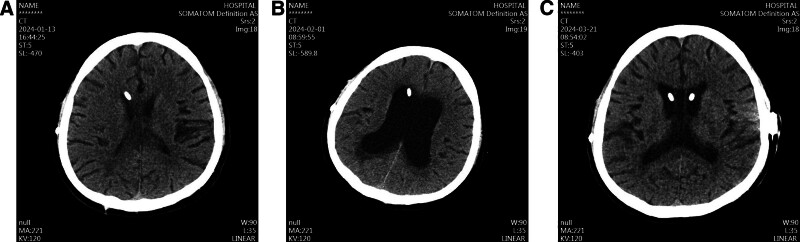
Representative CT scan images. (A) (January 13, 2024): This CT scan shows slit-like ventricles, indicating over-drainage. (B) (February 1, 2024): This CT scan shows significant ventricular enlargement, suggesting under-drainage. (C) (March 21, 2024): This CT scan, taken postoperatively after right-sided ventriculoperitoneal shunt surgery, shows normal ventricular morphology with appropriate drainage. CT = computed tomography.
